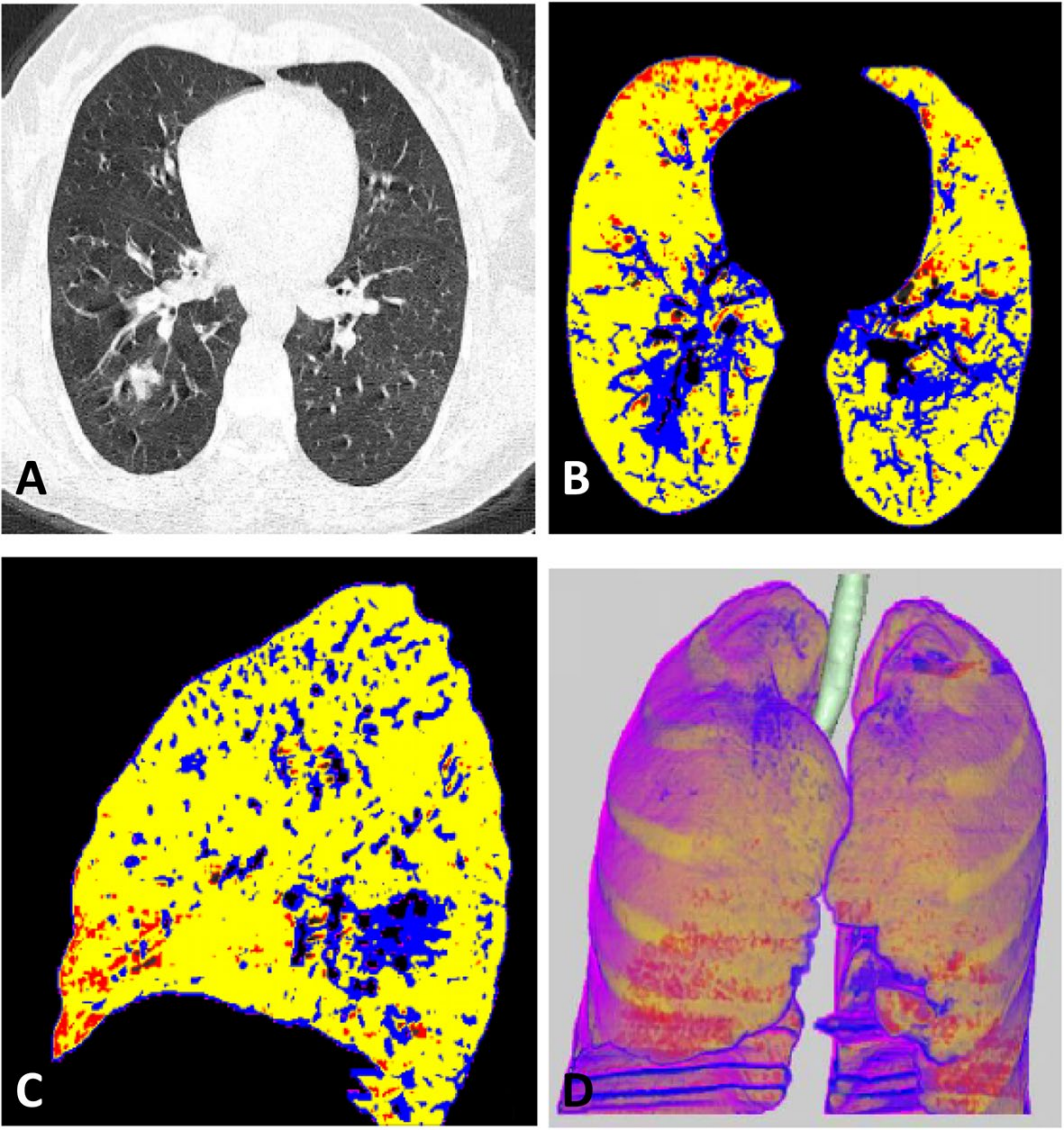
CT findings of female patient with mild disease, Total severity score (TSS) equal 4. A Axial CT image shows right lower lobe ground glass density. B and C Axial and sagittal colored analysis of the lung density revealed mild ground glass percentage (20%) in blue color compared to normal lung density which is represented with yellow color. D 3D image analysis of the lung with different densities
